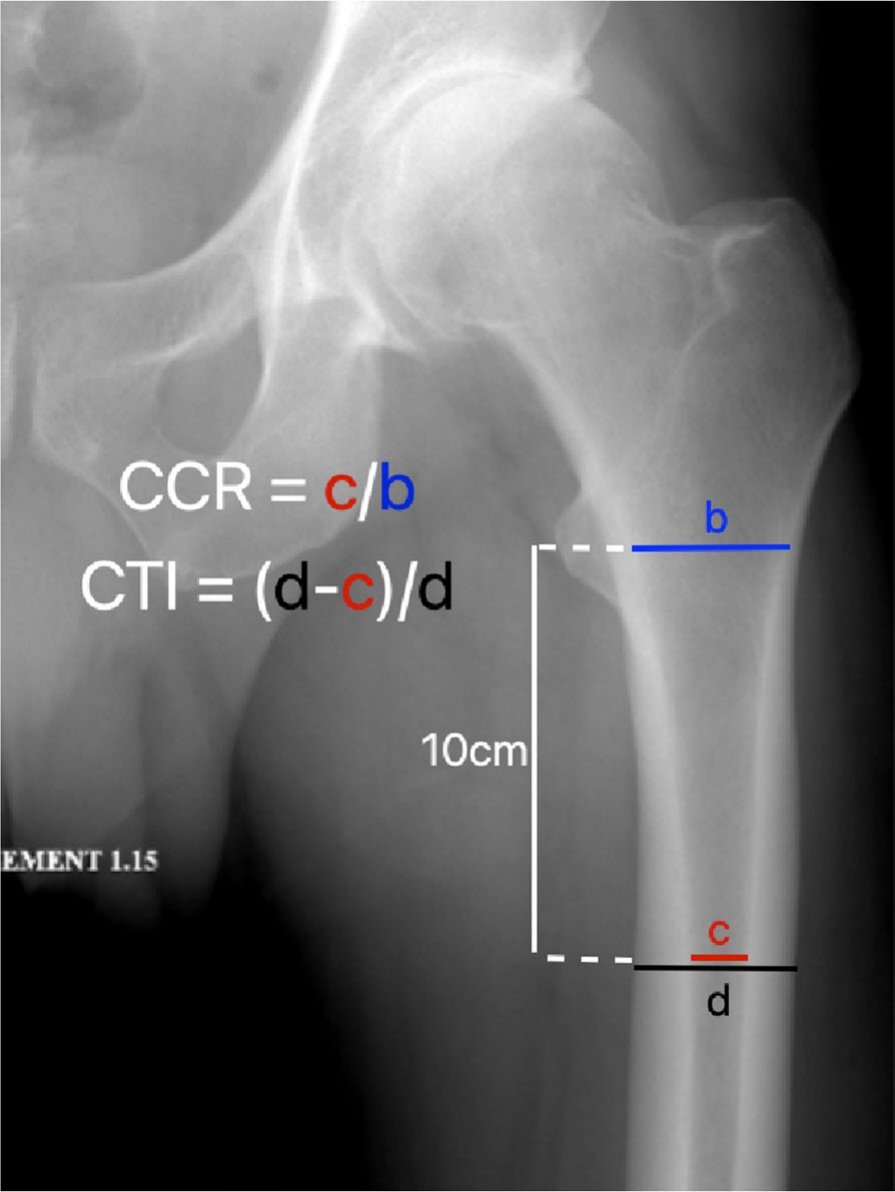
Cortical thickness index (CTI) and canal calcar ratio (CCR) were performed on preoperative anteroposterior pelvic radiographs, with CTI = (d – c) / d and CCR = c / b
